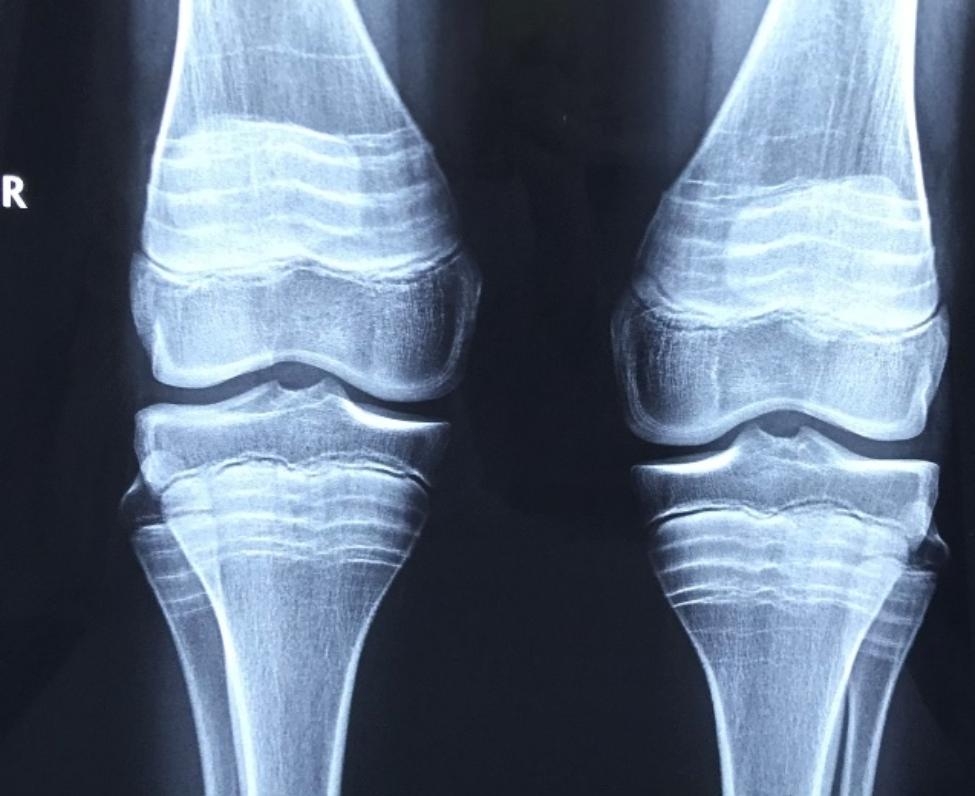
The radiograph of the knee joints of the proband (13 years old): the metaphyses are bell-shaped expanded, with transverse bands of sclerosis - likely resulting from intravenous bisphosphonates. The cortical layer is thinned. The structure of the bone tissue is sparse. The general structure of the joint is intact. The articular surfaces are congruent. Articular spaces are symmetrical. Growth zones are well defined
